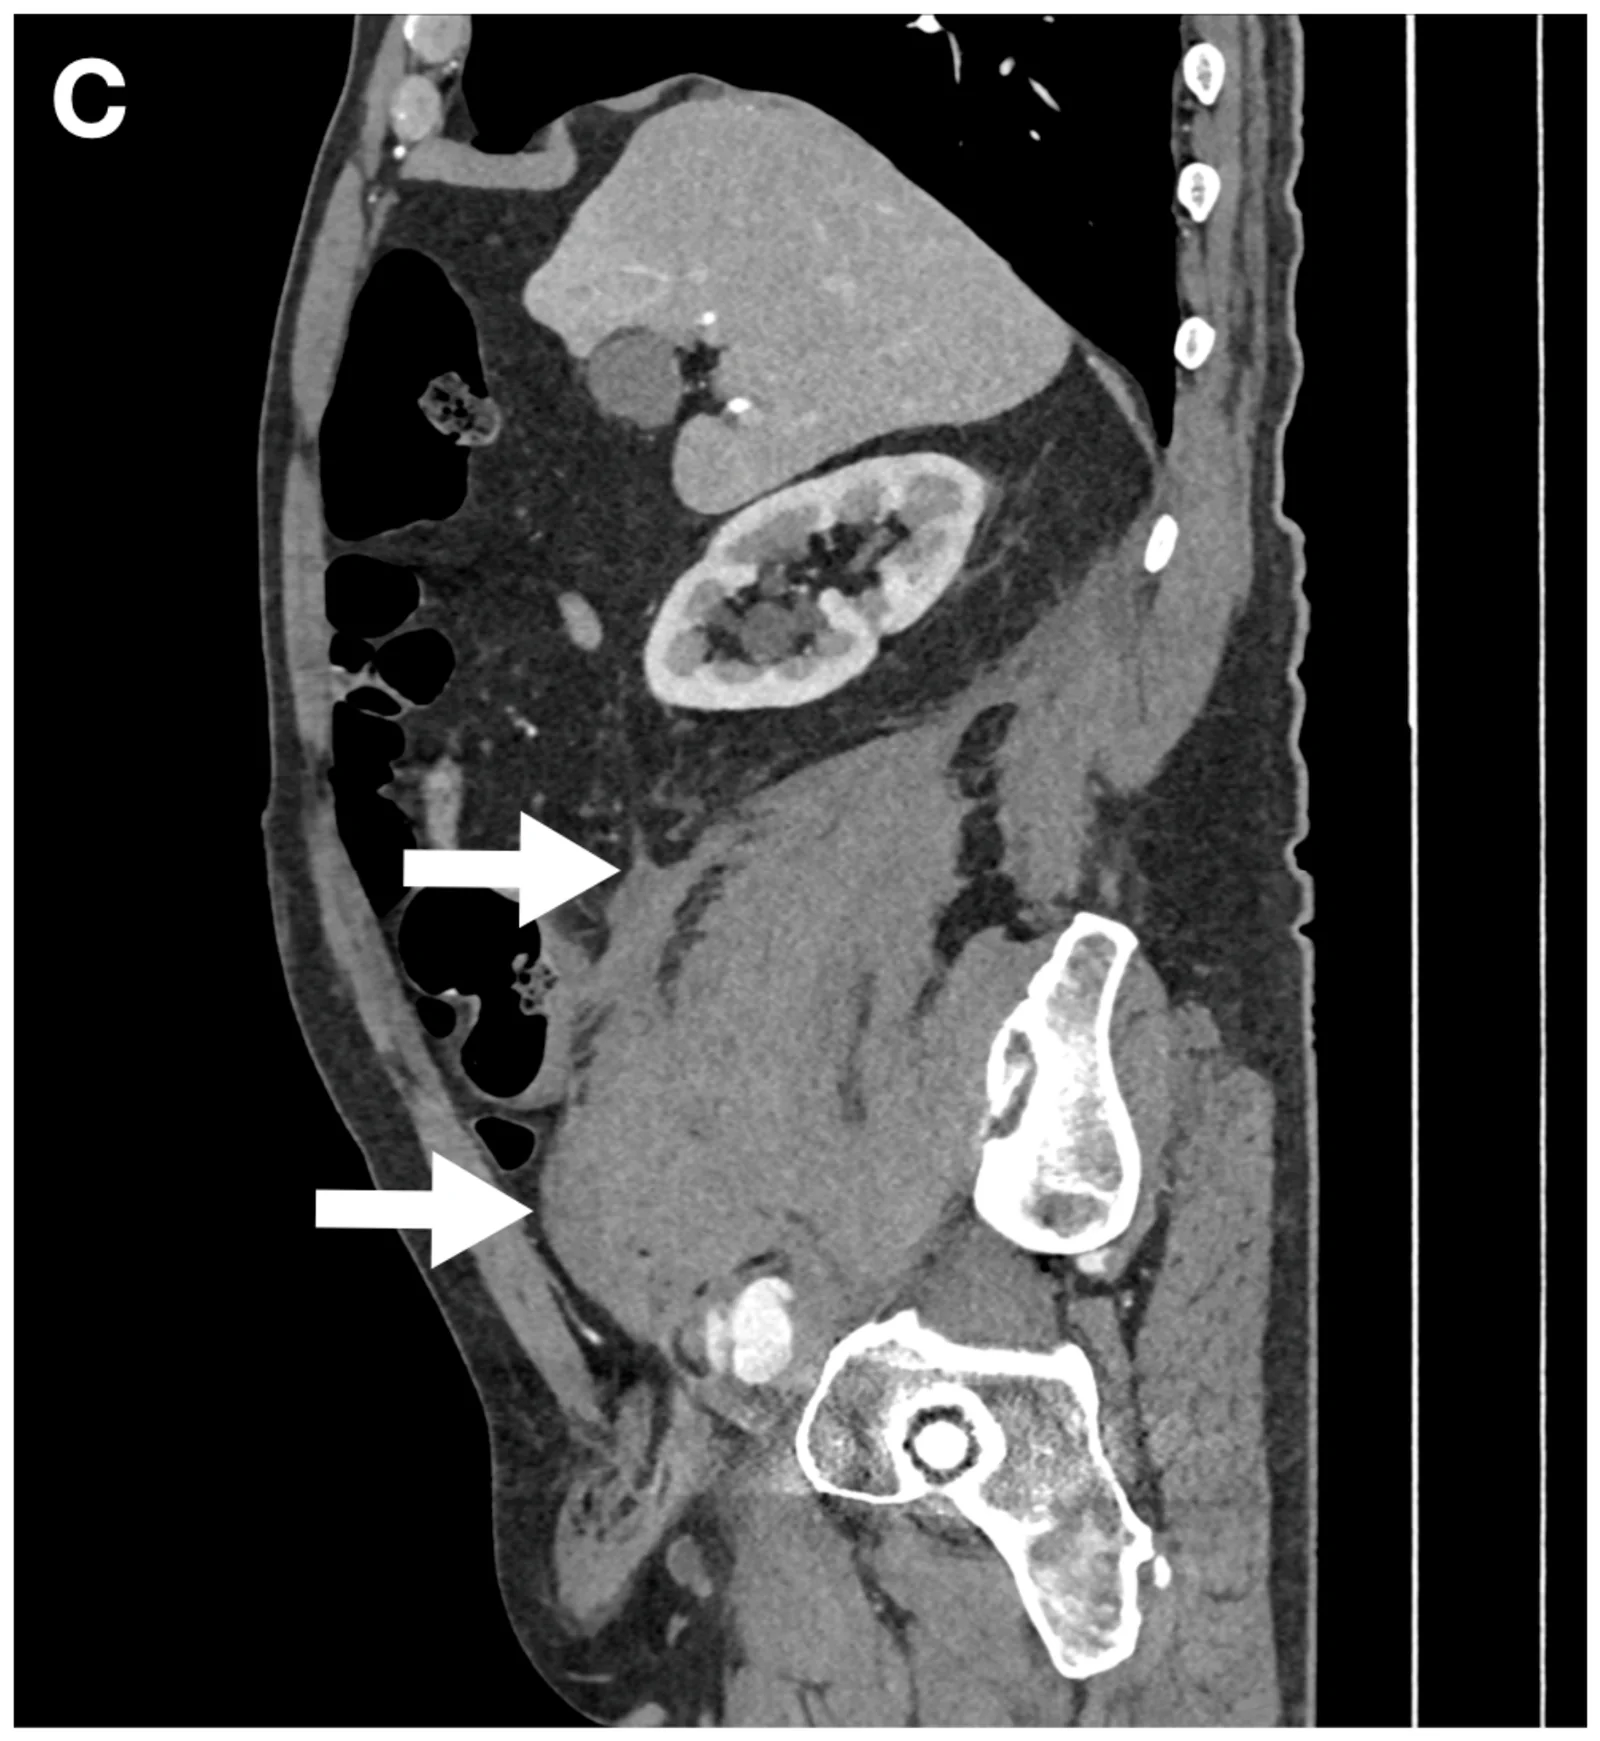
Computed tomography angiography (CTA) performed on the first postoperative day. (A) Axial arterial-phase CTA demonstrates active contrast extravasation arising from the right external iliac artery (arrow). (B) Coronal oblique/MIP reconstruction localizes the bleeding source along the right external iliac artery above the inguinal ligament at the level of inferior epigastric artery (arrow). (C) CTA shows the associated retroperitoneal hematoma extending along the right pelvic/iliac region (arrow). CTA enabled localization of the bleeding source and immediate endovascular treatment planning.
